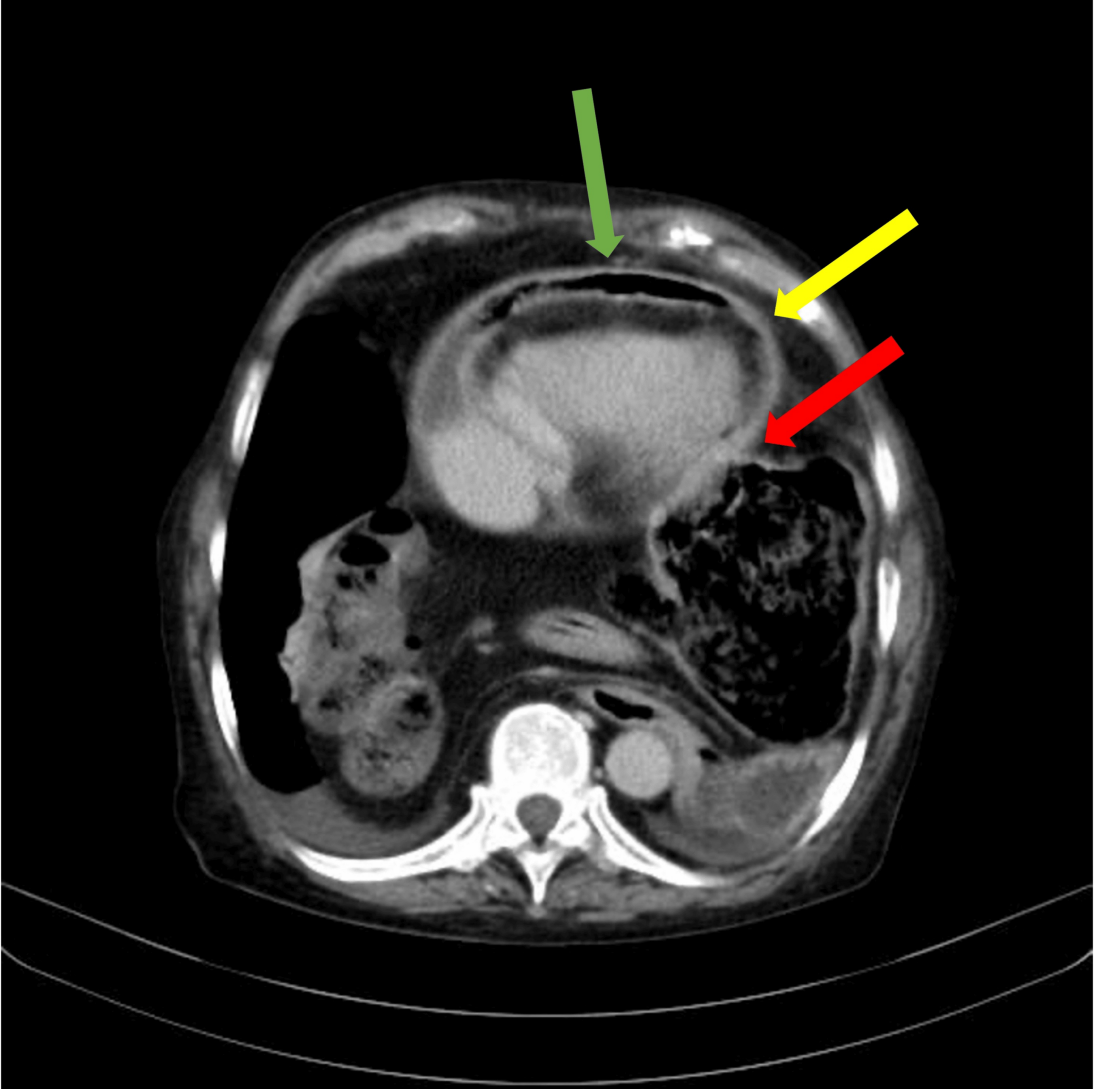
Chest CT, venous phase, 10th thoracic vertebra level, transversal plane The green arrow shows the pericardial effusion with gas. The thickened pericardium (yellow arrow) and the communication of the pericardium with the bowel (red arrow) are also visible.
